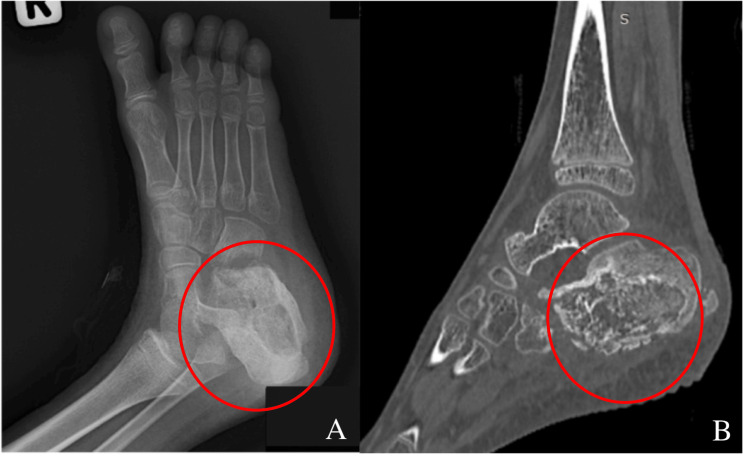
Preoperative radiograph of patient's right foot in oblique view (A) and CT image in sagittal plane (B) The lytic lesions and sequestrum, highlighted in red, show the bone destruction caused by osteomyelitis.
